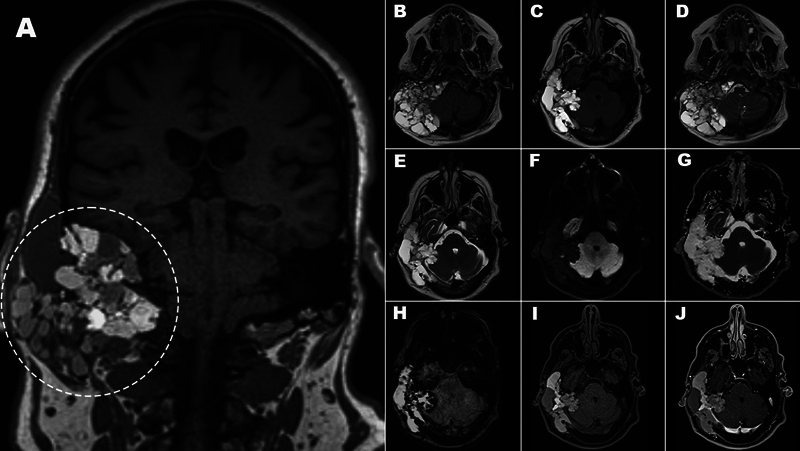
Brain magnetic resonance imaging dedicated to posterior fossa evaluation. Coronal T1-weighted non-contrast (
